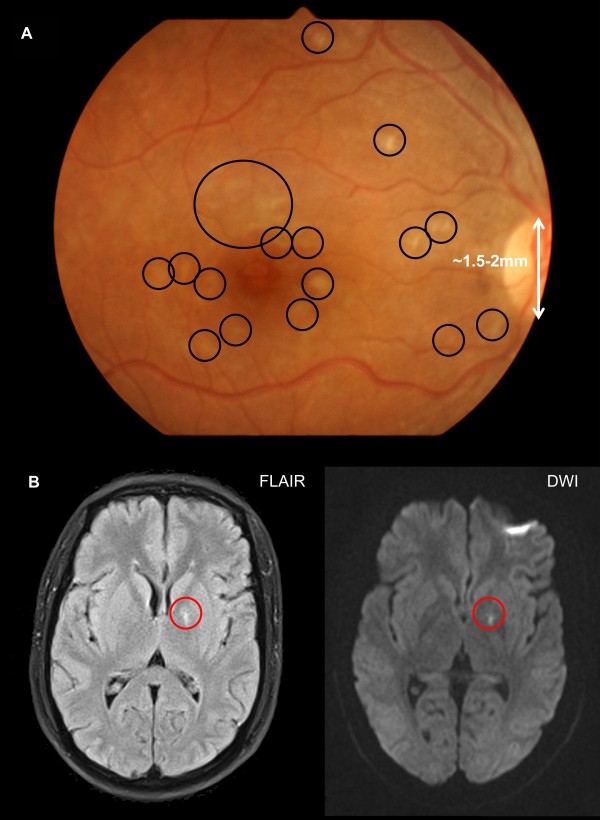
Retinal photograph and MRI from patient with cerebral malaria (GCS = 8 on enrollment), hyperlactaemia and 98% sequestered biomass. A the retina has typical lesions of retinal whitening (black circles) in the macula and fovea. Using the vertical optic disc diameter (white) as reference [12], each lesion of whitening is estimated at around 0.2-0.5 mm diameter. B on MRI the only abnormality was high signal in the globus pallidus on T2/FLAIR (left) and DWI (right).
